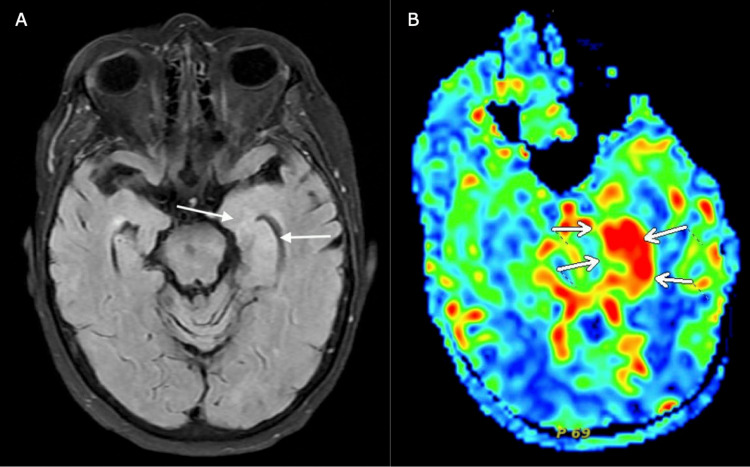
(A) MRI of the brain T2/fluid-attenuated inversion recovery (FLAIR) sequencing showing left mesial temporal lobe edema with contrast enhancement. (B) MRI of the brain showing increased cerebral blood flow in the same area.
